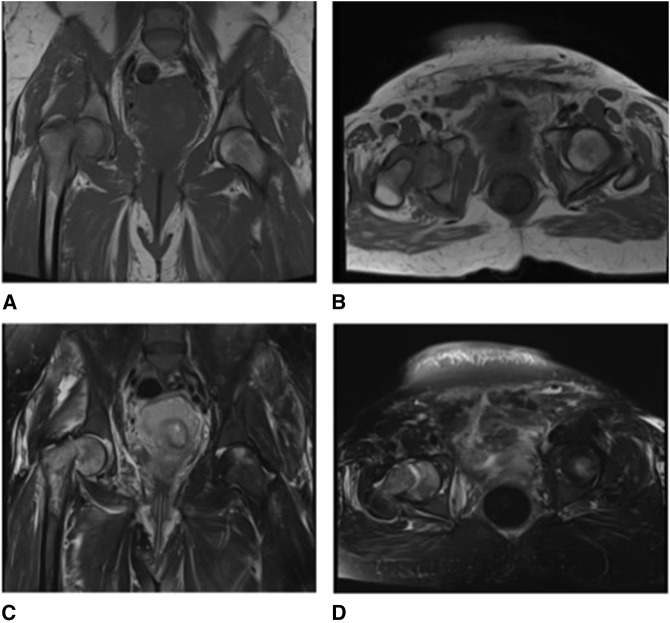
T1 coronal view (A), T1 axial view (B), T2 coronal view (C), and T2 axial view (D) of pelvic MRI showing a displaced right femoral neck fracture and evidence of early osteonecrosis in the left femoral head.
